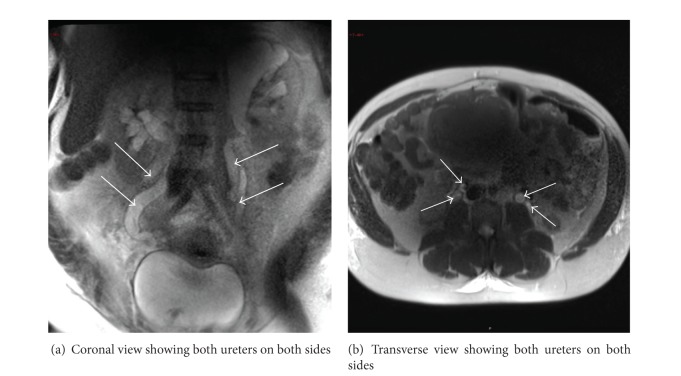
Abdominal Magnetic resonance imaging picture of the patient at day 2 post cesarean section.
